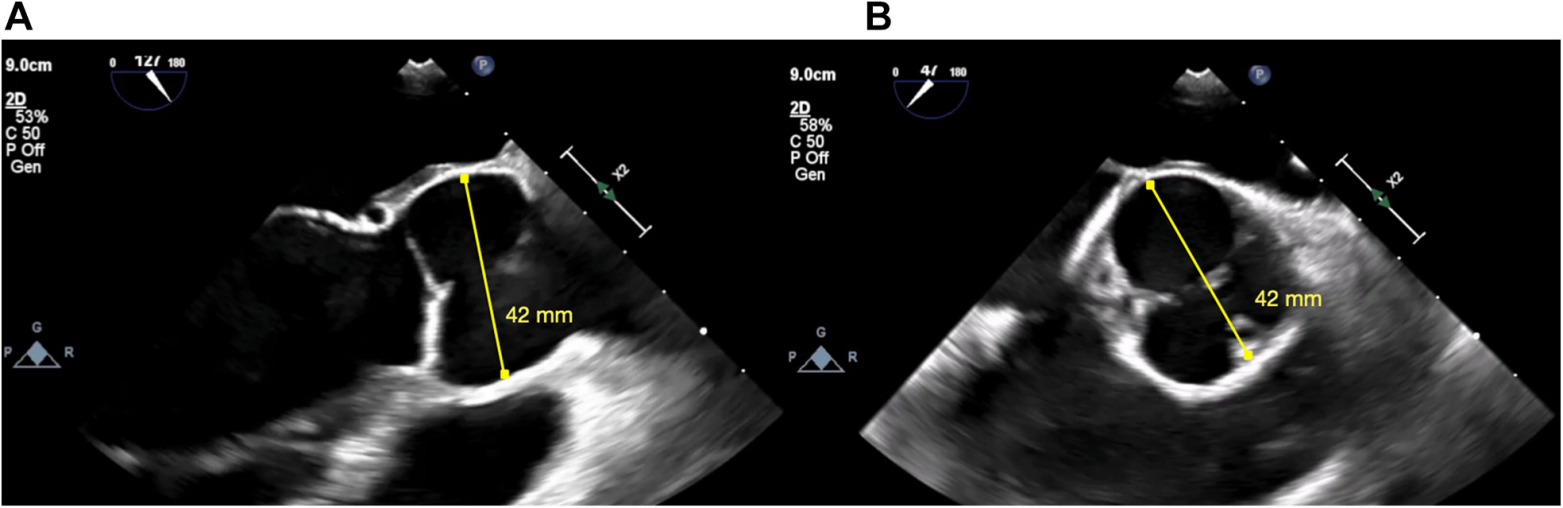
Aortic Root Diameter on Transesophageal Echocardiography (A) Midesophageal long-axis view and (B) midesophageal aortic valve short-axis view with a maximum aortic root diameter of 42 mm. Abbreviations as in Figure 1.
